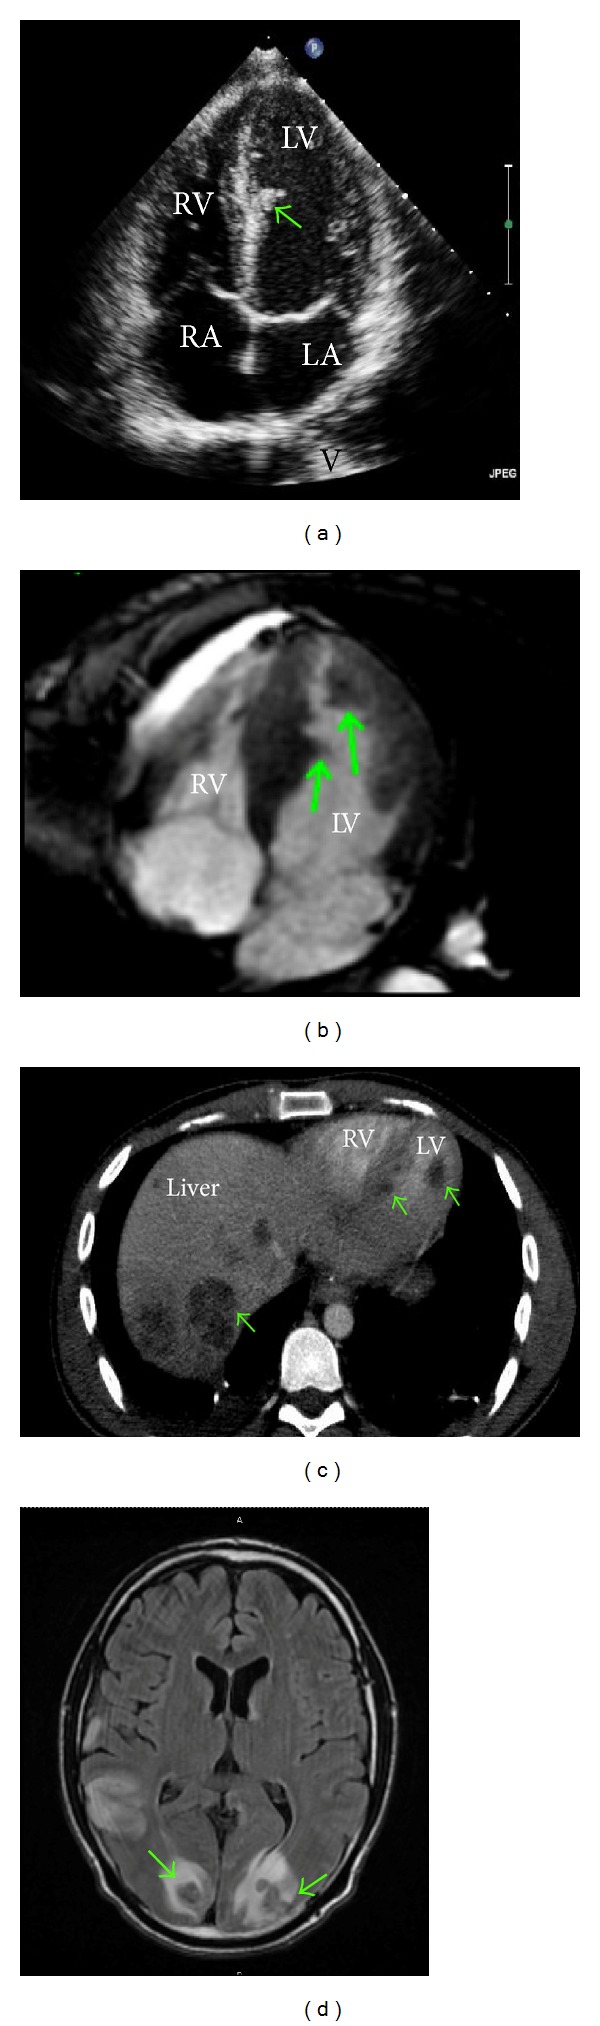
(a) An apical 4-chamber view of 2D echo showing mass in left ventricular cavity attached to left side of interventricular septum. (b) Cardiac MRI showing masses in left ventricular cavity attached to the interventricular septum and lateral wall. (c) CT chest and abdomen showing left ventricular masses and metastatic liver lesions. (d) MRI brain showing bilateral occipital lobe metastatic lesions surrounded by vasogenic edema.
